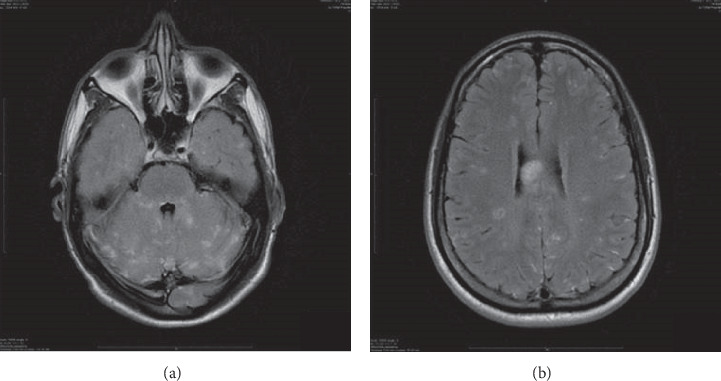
MRI brain (T2 axial section). Extensive postcontrast T2 FLAIR enhancement of periventricular and cerebellar diseases with patchy leptomeningeal enhancement accentuated by the FLAIR technique. (a) Large T2 FLAIR signal in the body of corpus (snowball lesions) and throughout the periventricular white matter. (b) Regions of T2 FLAIR signal correlated with DWI (b1000) diffusion restriction.
